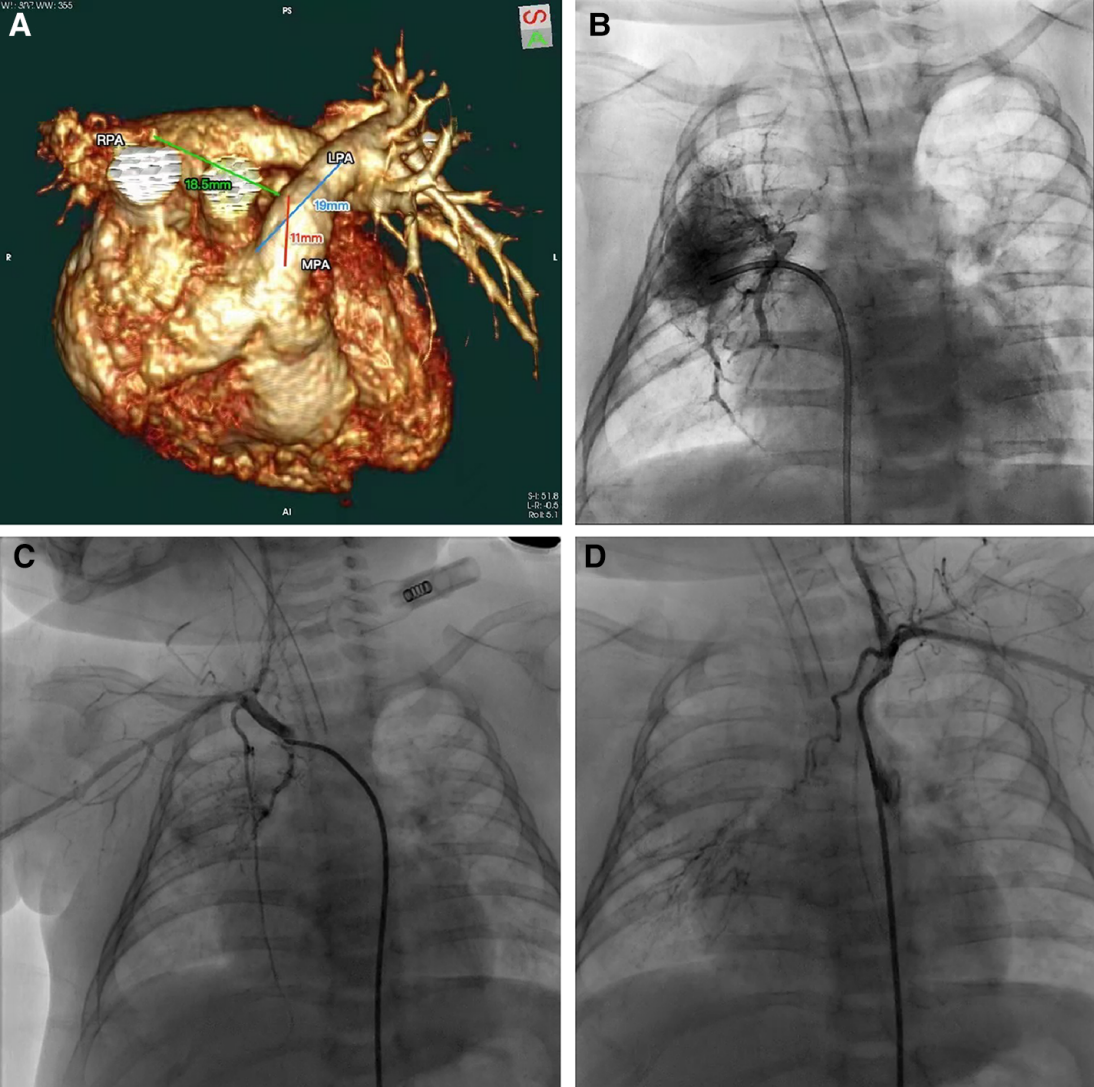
(A) CTA and three-dimensional reconstruction revealed the absence of intrapericardial right pulmonary artery. (B–D) Cardiac catheterization revealed blood supply of upper right pulmonary artery comes from right subclavian artery and inferior right pulmonary artery was supplied by left subclavian artery.
